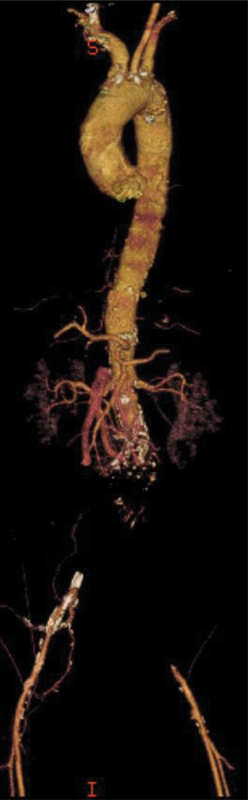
Three-dimensional computed tomography angiography reformat showing acute occlusion of the infrarenal aorta with distal reconstitution in the iliac arteries.
